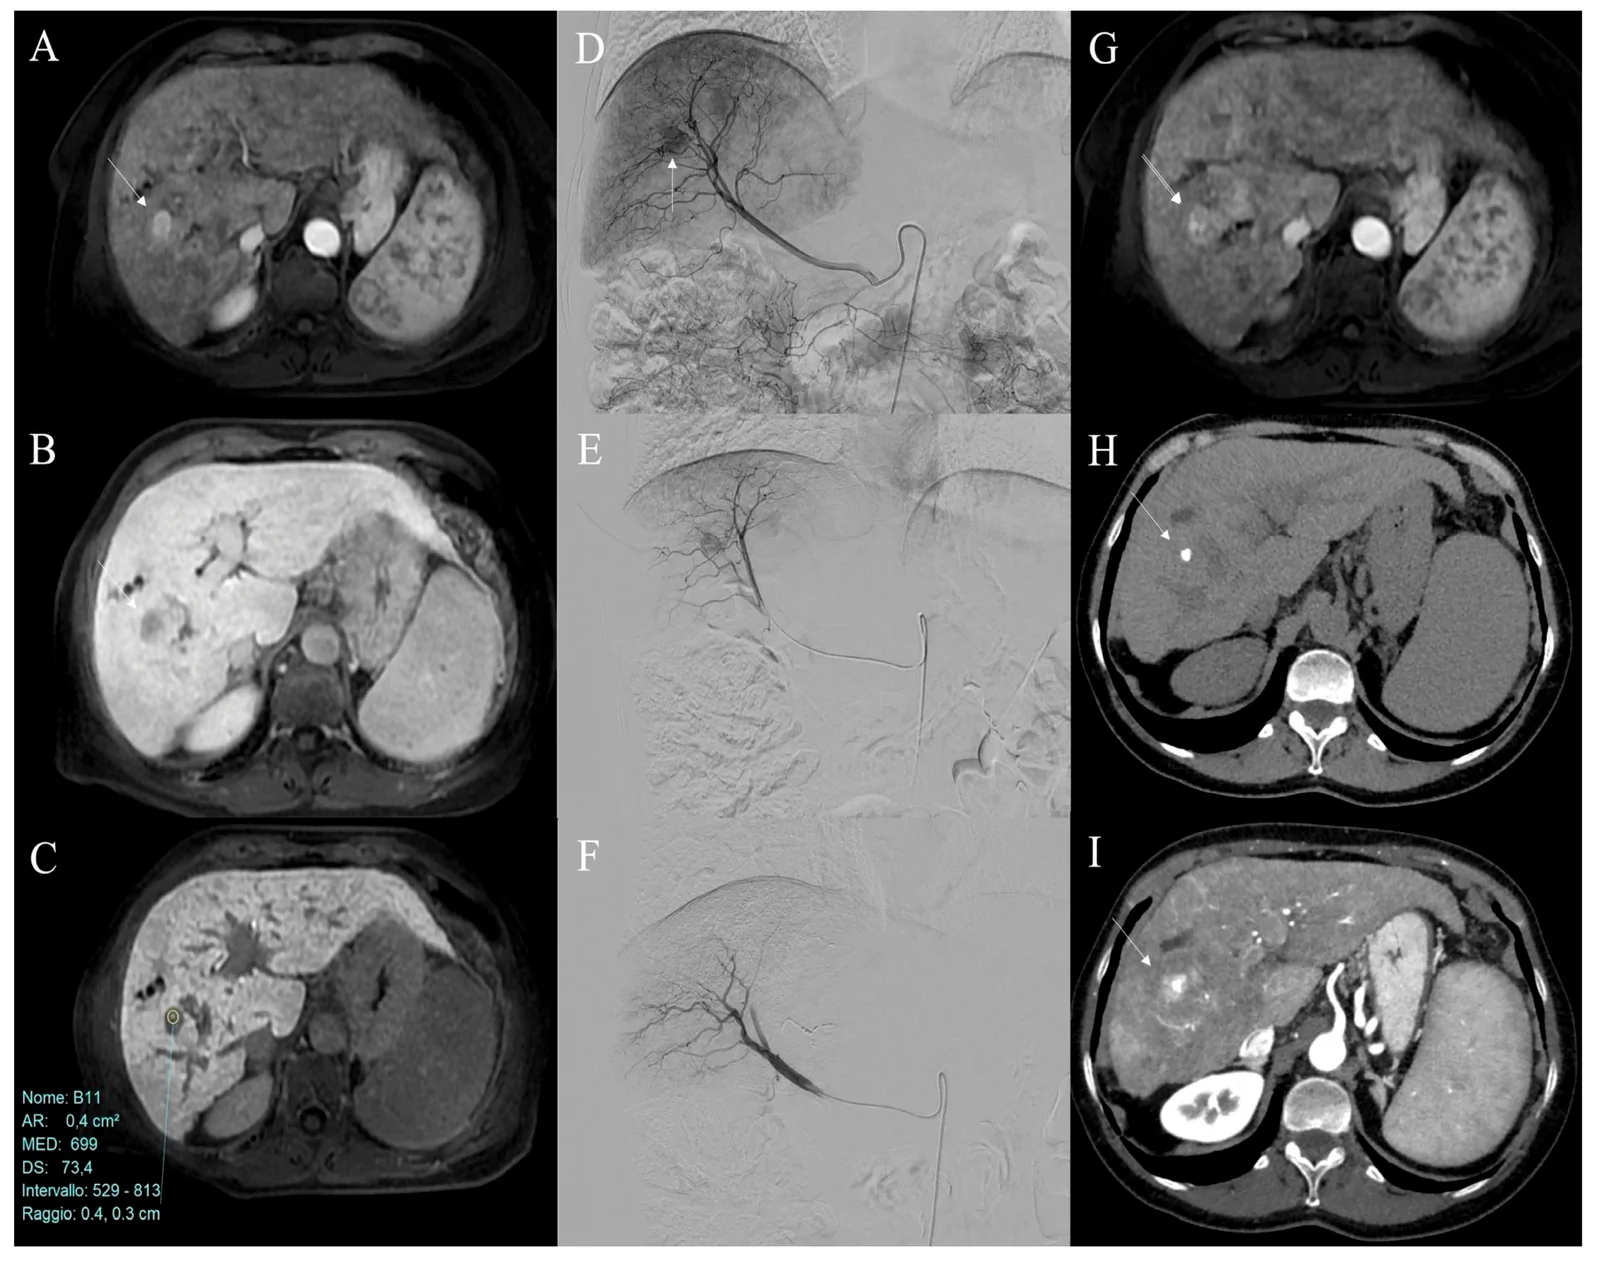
Axial contrast-enhanced MRI images acquired with a hepatospecific contrast agent showing a 14 mm HCC nodule located in segment 8, with arterial phase hyperenhancement (A), washout in the portal-delayed phase (B), and mild signal hypointensity (mean = 699) in the HBP (C). Angiographic images documenting the cTACE procedure with identification of the nodule (D), injection of chemotherapeutic agents and embolic material (E), and subsequent confirmation of the absence of tumor vascularization at the end of the procedure (F). At 6-month follow-up, an axial arterial-phase MRI image (G) demonstrates persistence of disease and a diameter increase (18 mm vs. 14 mm), resulting in PD according to mRECIST criteria. Non-contrast (H) and arterial-phase (I) axial CT images obtained at 6-month follow-up show accumulation of iodized oil (Lipiodol-Ultrafluid, LUF) at the site of the treated nodule, with persistence of disease at its periphery.
